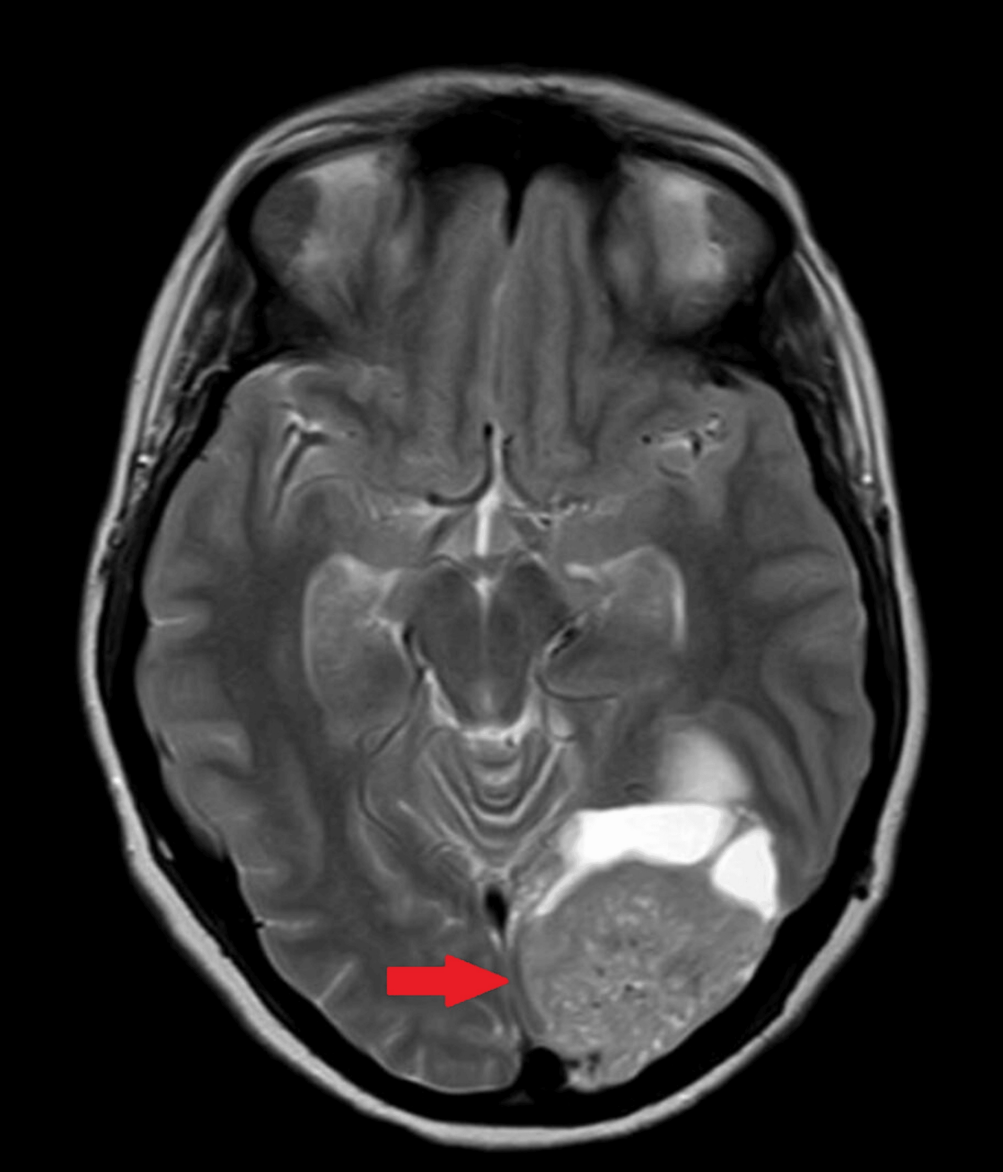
Preoperative axial T2-weighted MRI brain scan showing left occipital solid-cystic mass with midline shift and inferior extension (red arrow).
